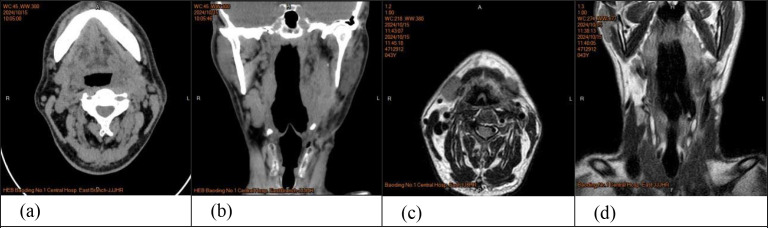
(a, b) Neck CT at two months after surgery: The soft tissue of the left lateral wall of the oropharynx was slightly thickened, the structure was less regular, and the surrounding fat space was blurred. The shape of the laryngeal cavity was regular and symmetrical, and the pharyngeal recess, pyriform sinus, and epiglottis valley were present. The epiglottic cartilage, thyroid cartilage and cricoid cartilage were regular in shape. There was no obvious abnormal density shadow in either lobes of the thyroid gland, and the trachea was centered. (c, d) Neck MRI at two months after surgery: The local subcutaneous fat space in the left submandibular area was blurred, and the patchy T2 fat-suppression high signal was seen. The rest of the neck structure was still clear, and no obvious abnormal signal was found.
